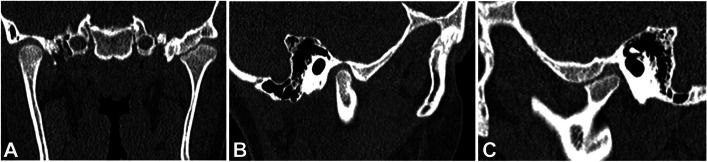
Images of maxillofacial computed tomography (CT) showing both TMJs in coronal view (A), right TMJ in sagittal view (B) and left TMJ in sagittal view which showed the mandibular condyle significantly erosed (C)
